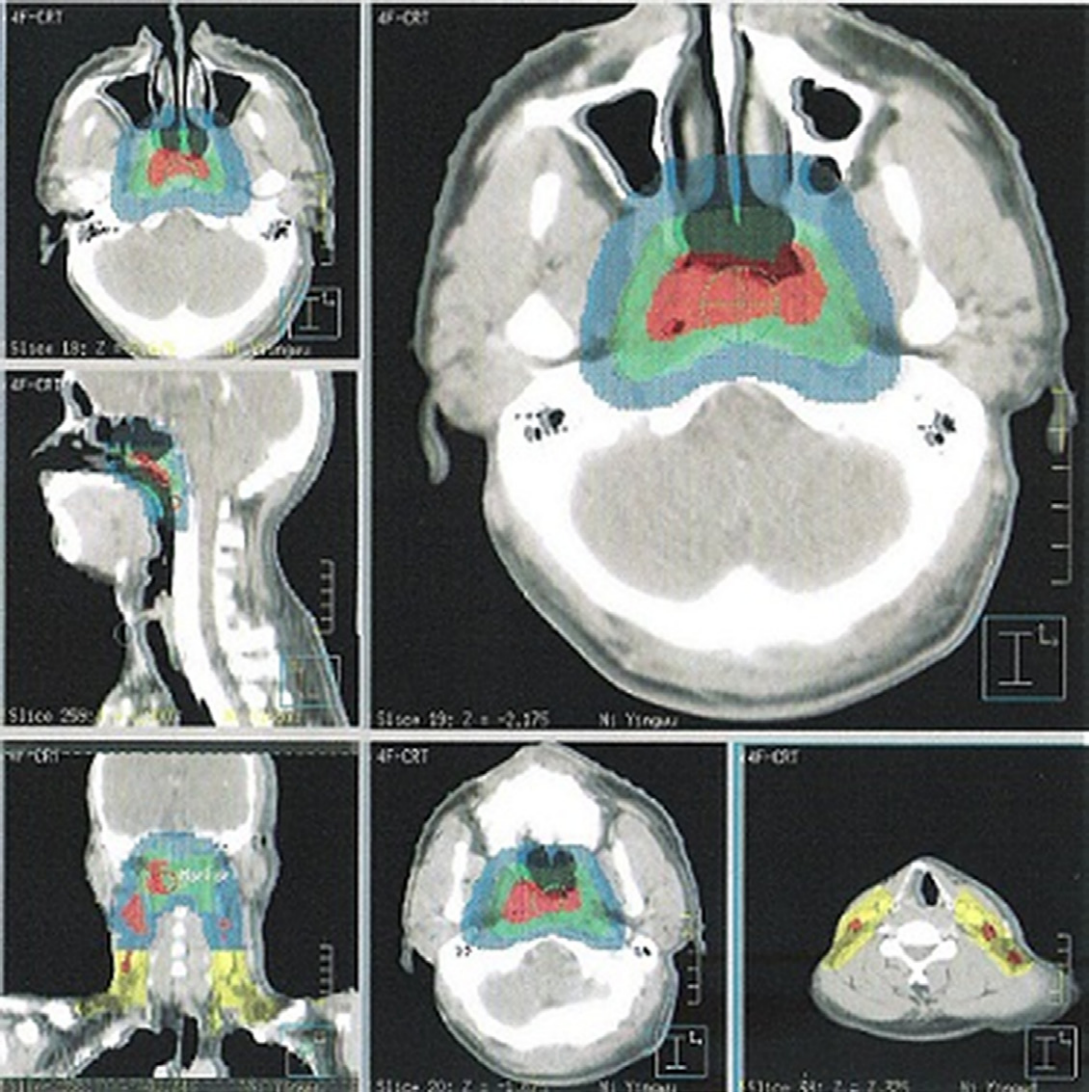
Selected CT slides for demonstrating the delineation of target volumes (Red area: PGTVnx and GTVnd; Green area: PTVnx; Blue area: PTV1; Yellow area: PTV2).
